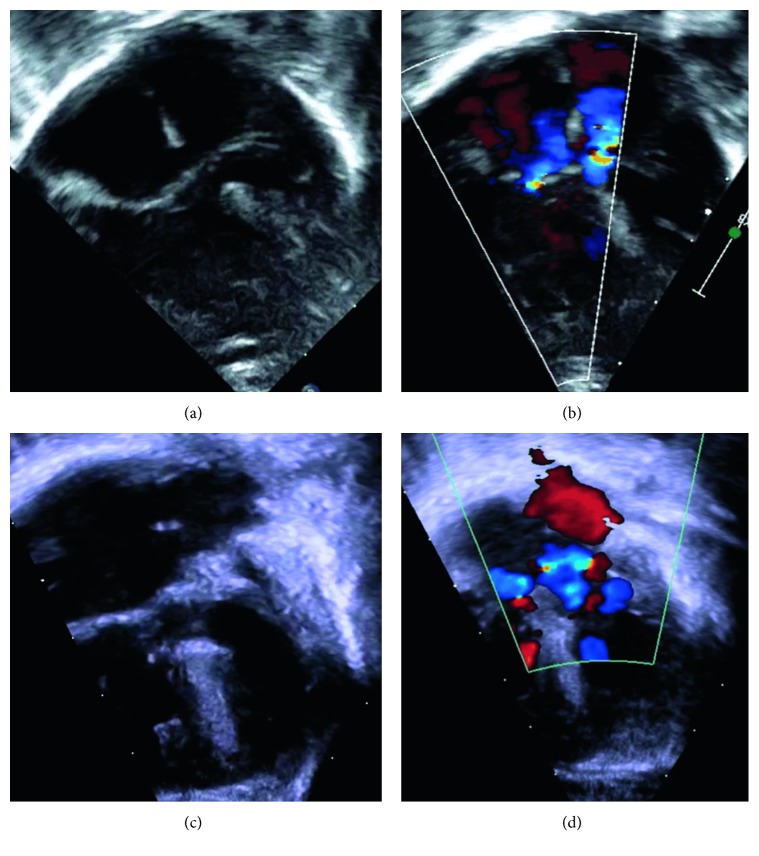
Postnatal four-chamber cardiac images. (a) Twin A 2D imaging showing a ventricular septal defect, primum atrial septal defect, and secundum atrial septal defect. (b) Twin A color Doppler showing left, central, and right atrioventricular valve regurgitation (blue flashes moving away from the transducer. (c) Twin B 2D imaging showing a ventricular septal defect, primum atrial septal defect, and secundum atrial septal defect. (d) Twin B color Doppler showing left and right atrioventricular valve regurgitation (blue flashes moving away from the transducer).
